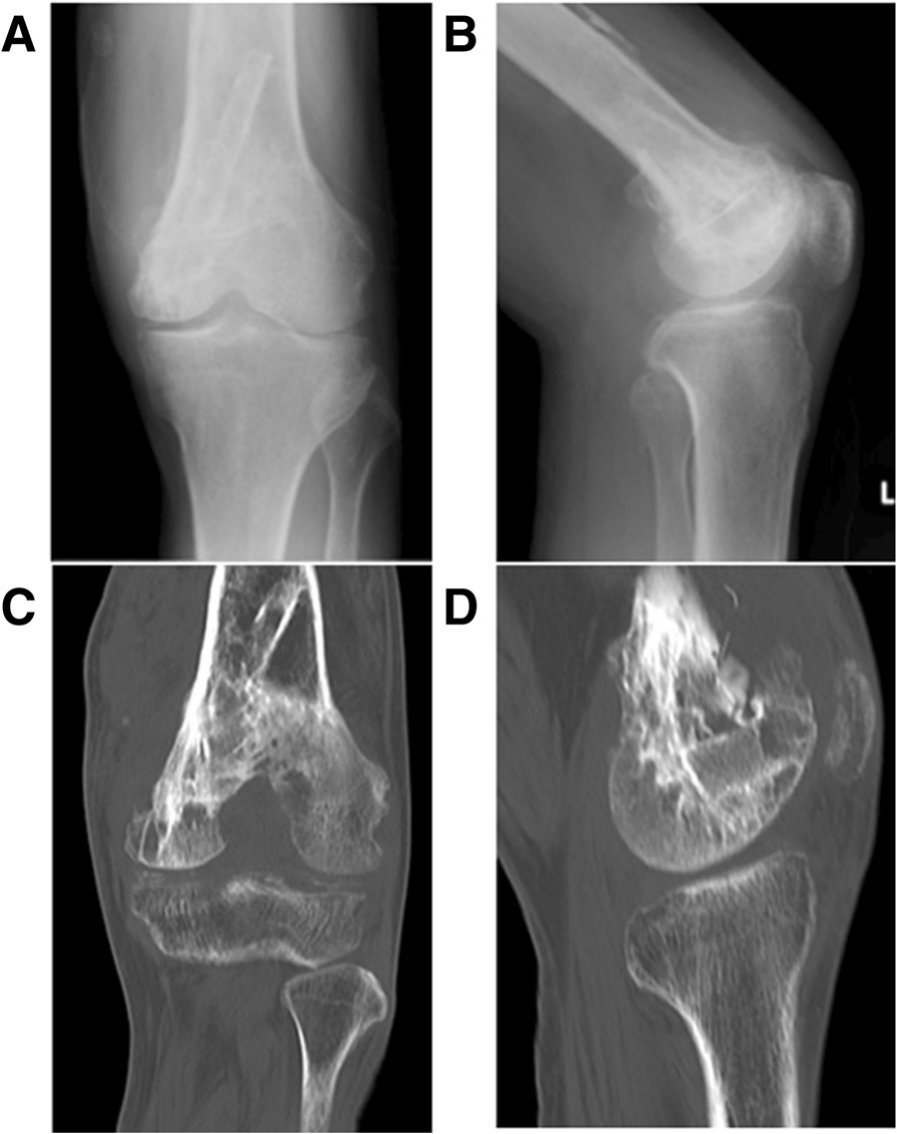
Plain X-ray findings and computed tomography findings. Plain X-ray (a, b) and computed tomography (c, d) show bone grafts, including a fibula graft performed at the time of initial surgery involving transplantation of the femoral metaphysis to the epiphyseal area. Neither bone translucency nor destruction is apparent on these images
